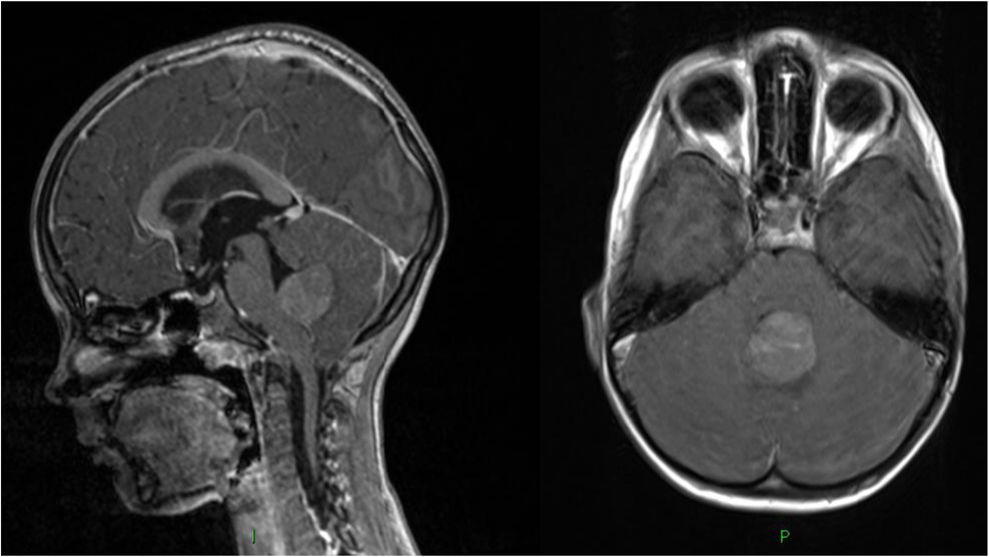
MRI brain at presentation
